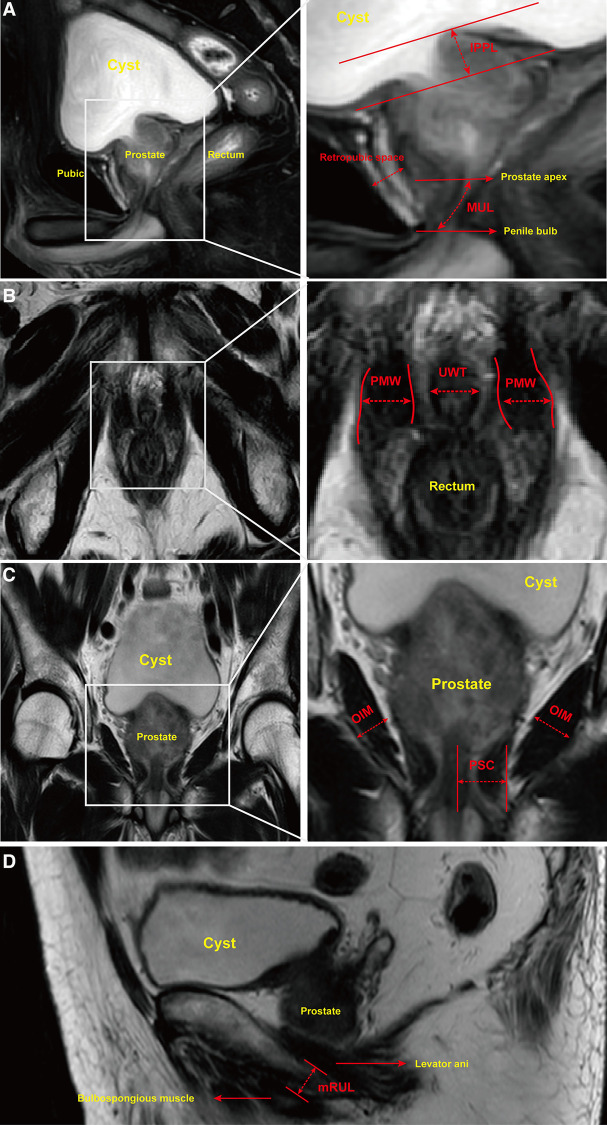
Urethral and pelvic anatomic parameters measured on MRI. Notes: (A) Sagittal T2-weighted sequences allowed for membranous urethral length (MUL) to be measured from inferior edge of the prostate apex to the superior margin of the penile bulb. Intravesical prostatic protrusion length (IPPL) was measured by the vertical distance from the tip of the protruding prostate to the base of bladder. Retropubic space was measured by the shortest distance between posterior margin of pubis and anterior of prostate. (B) Axial T2-weighted sequences allowed for puborectalis muscle width (PMW) and urethral wall thickness (UWT) to be measured. PMW was measured by the distance between inner and outer side of puborectalis muscle at the thickest portion. (C) Coronal T2-weighted sequences were used to measure peri-urethral sphincter complex (PSC) thickness and obturator internus muscle (OIM). (D) Minimal residual membranous urethral length (mRUL) was measured from the lower margin of the puborectalis muscle to the upper margin of the bulbospongiosus muscle in a direction parallel with the membranous urethra on Sagittal T2-weighted sequences.
